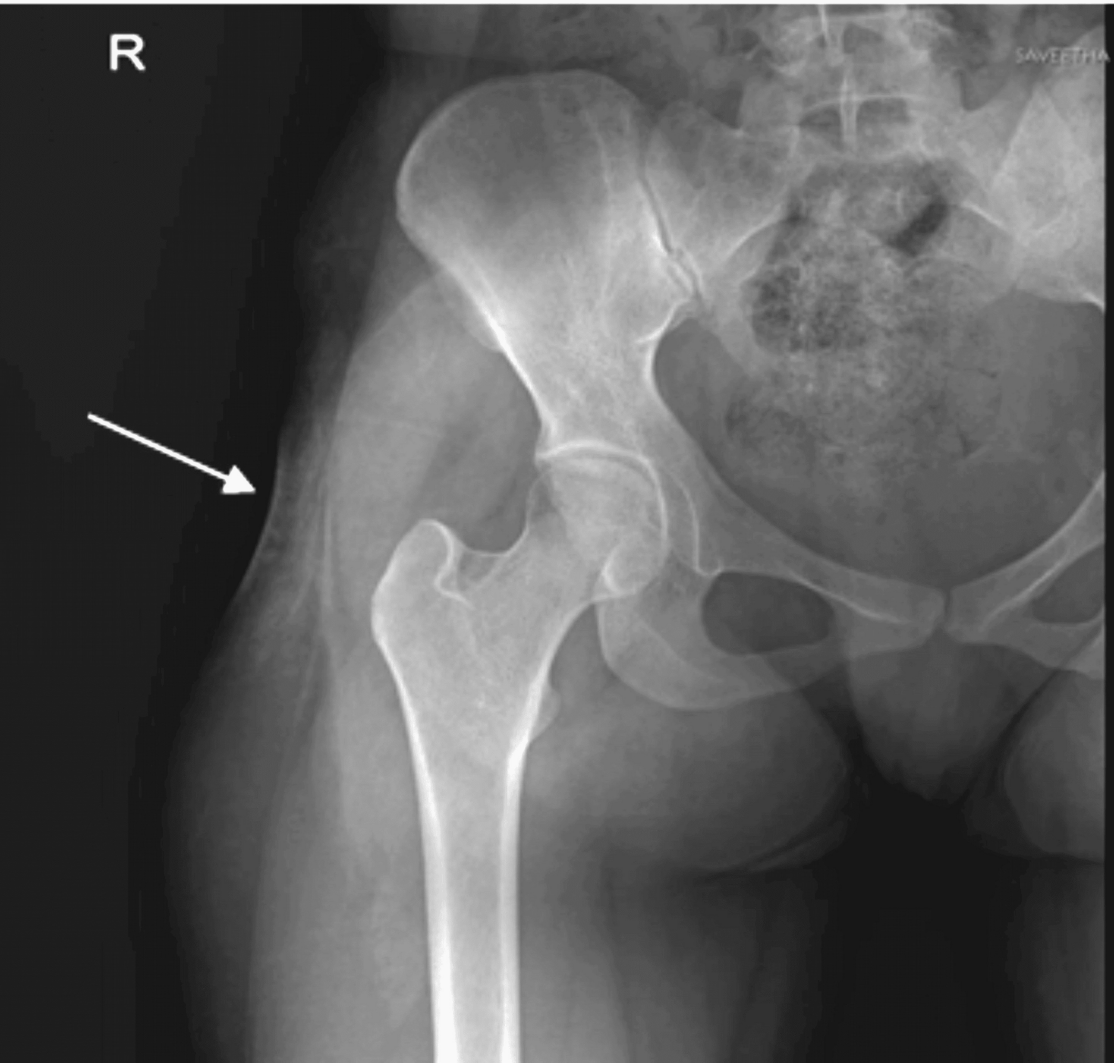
Radiograph of the right hip joint showing superficial soft tissue concavity in the lateral aspect with a focal increase in radiodensity.
